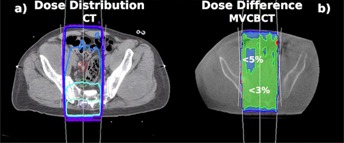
Dose difference for a pelvis plan: (a) the CT planned dose distribution and (b) relative dose difference planned on a 13.5 MU exposure eFOV MV CBCT of a pelvis patient. The treatment plan consisted of opposed AP/PA, with a one‐third to two‐thirds beam weighting.
